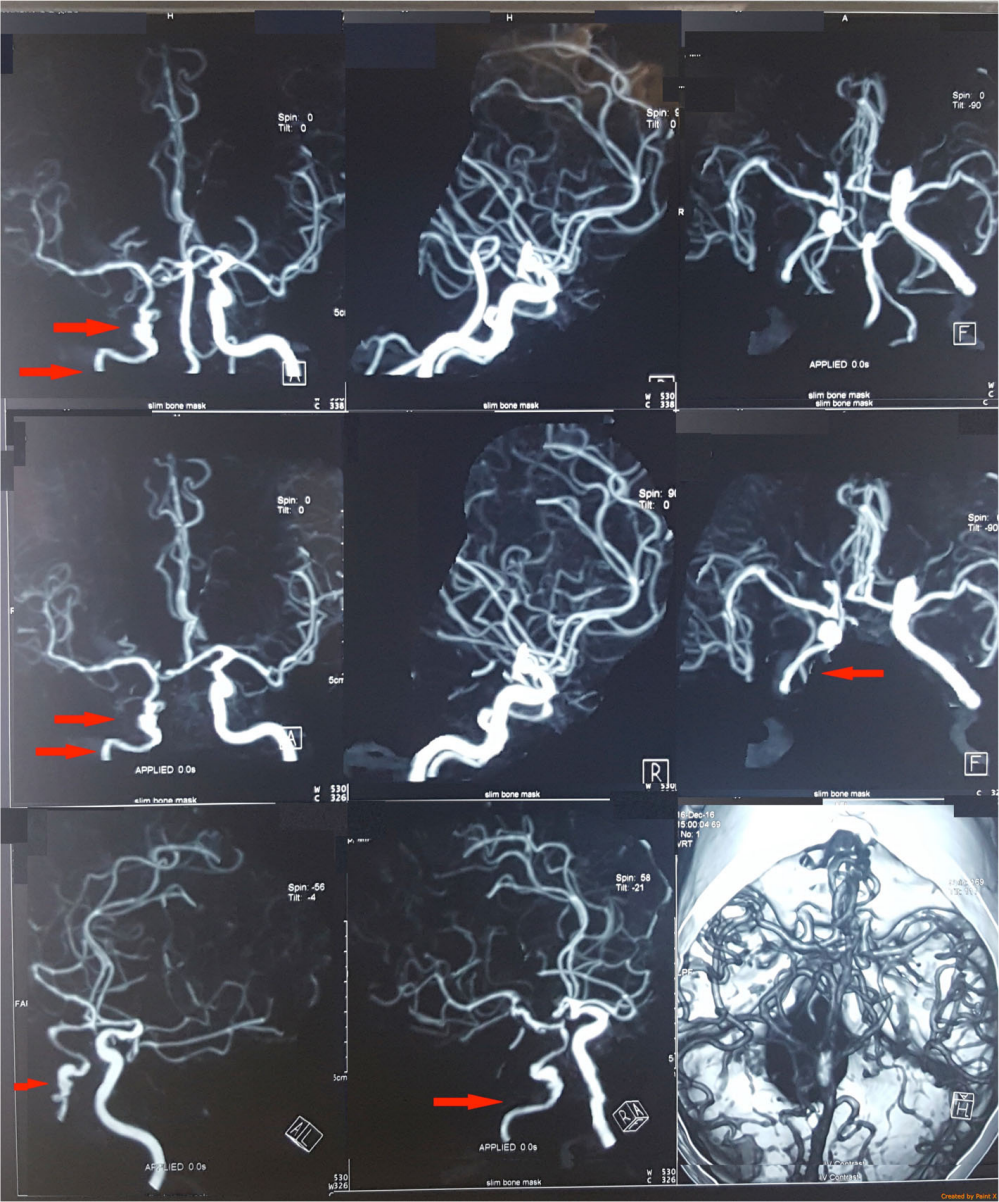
CT cerebral angiogram showing generalized caliber reduction of right internal carotid artery (Shown by *red arrow*)
